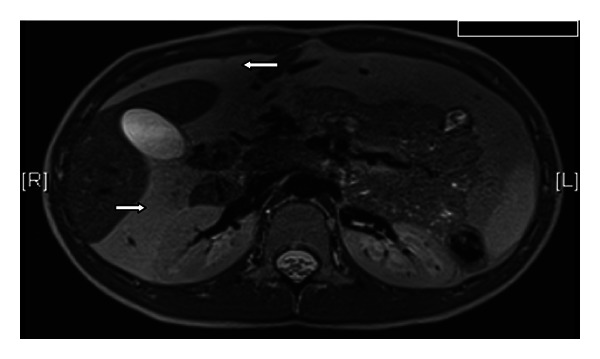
MRI abdomen: lipomatous tissue anterior to the liver and between the right lobe of the liver and the right kidney.
